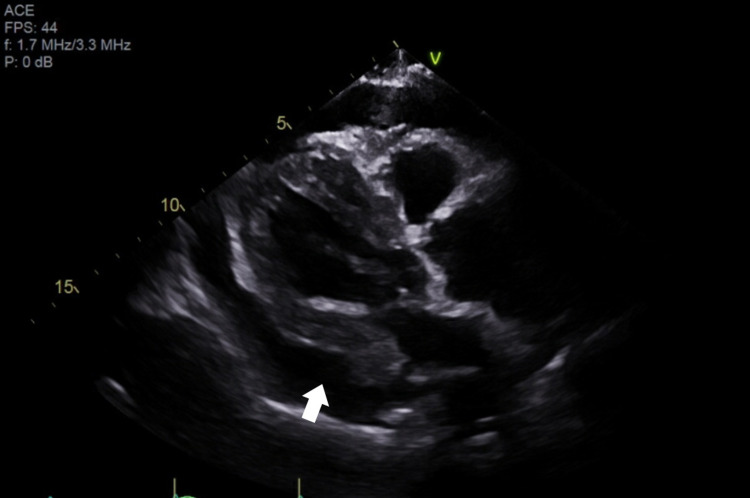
Long parasternal view of the heart on TTE showing large pericardial effusion. TTE: transthoracic echocardiography
